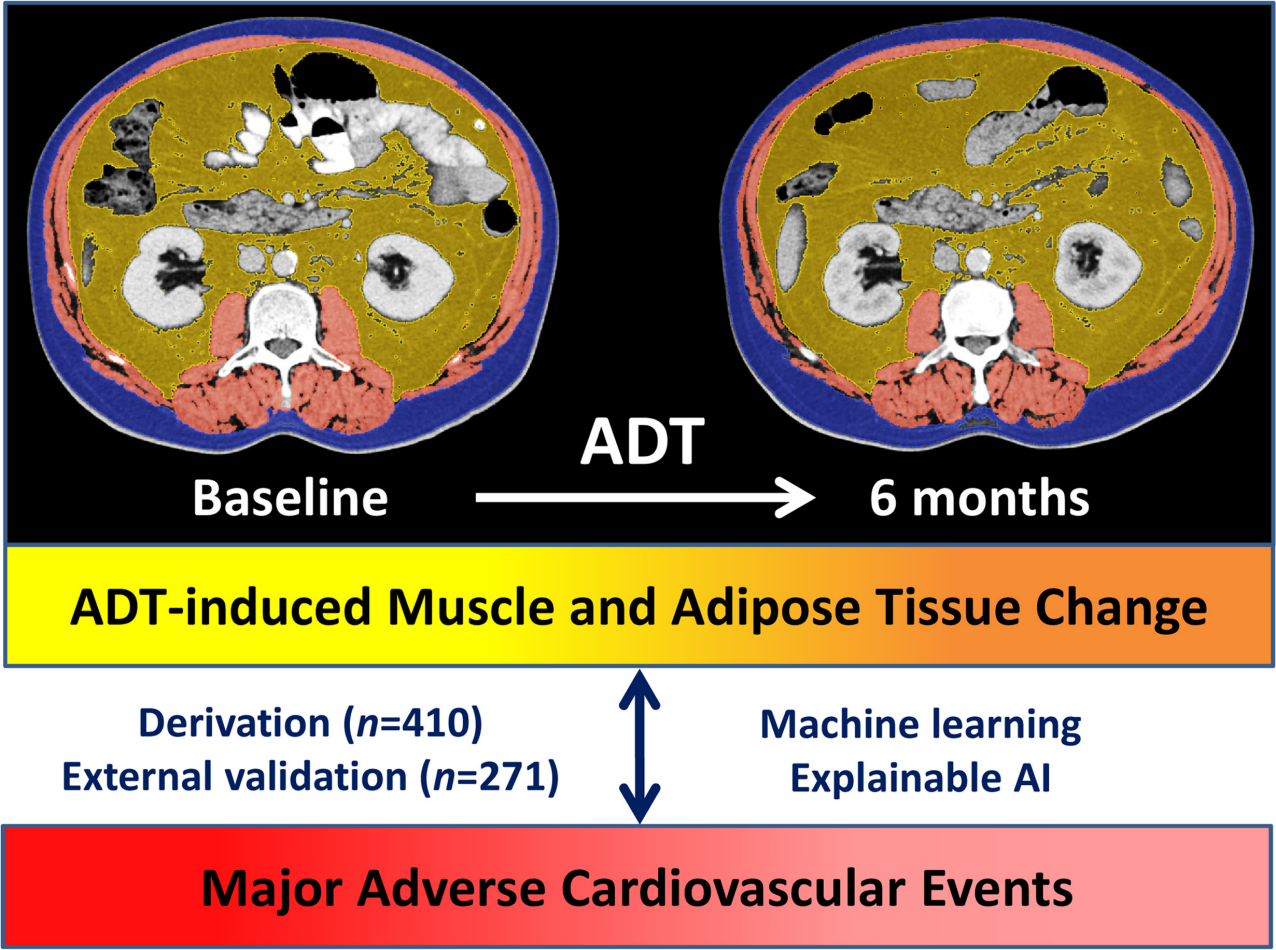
Utilization of explainable AI to explore the relationship of ADT‐induced body composition change with major adverse cardiovascular event. Body composition was assessed on a transversal computed tomography slice at the level of L3. ADT, androgen deprivation therapy; AI, artificial intelligence.
